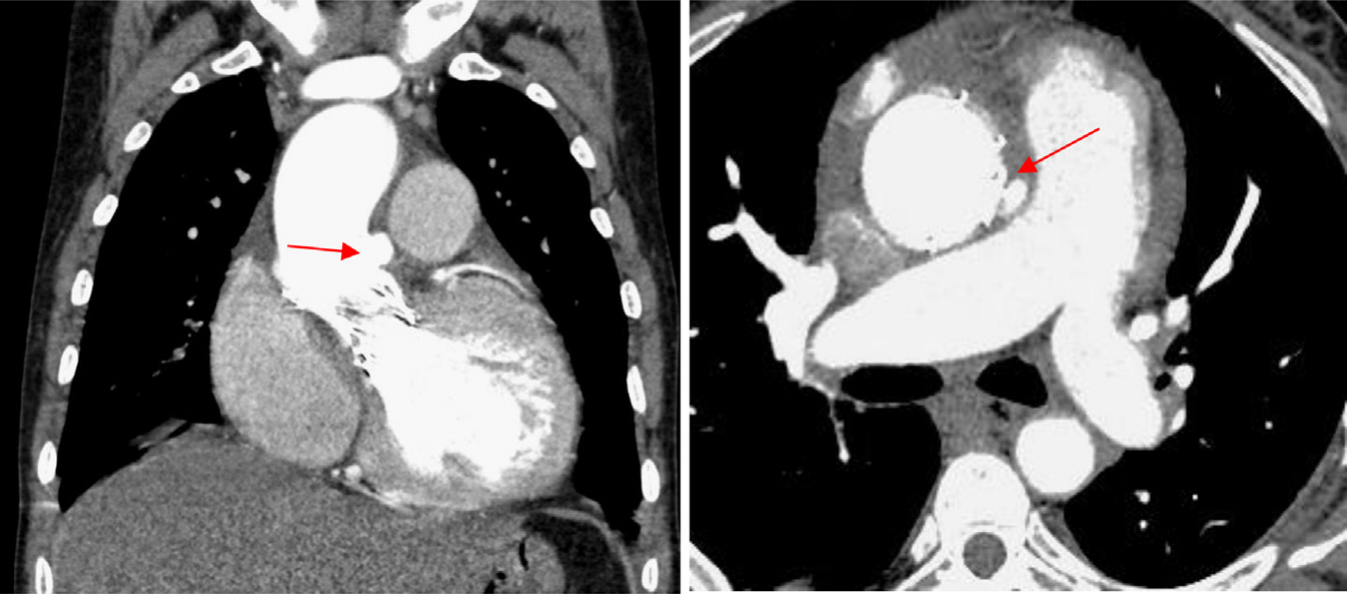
Computed Tomography Scan (A) Perivalvular mycotic aneurysm seen in aorta. (B) Axial section showing aortic mycotic aneurysm with a small neck (red arrow indicating the lesion).
